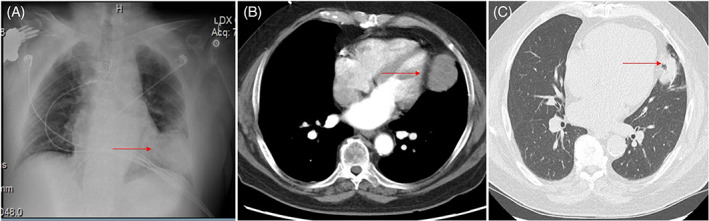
(A) Chest X‐ray showing a rounded mass measuring approximately 8 cm in left lower lobe. (B) CT chest showed a 6.6 cm enhancing soft tissue mass within the left lower mediastinum. A focal point measuring approximately 1 cm can be seen where there does not appear to be a fat plane of separation from the left ventricular myocardium. (C) CT heart angiogram showed a 6.9 by 5.4 cm mass in left inferior mediastinum with a stalk‐like connection to pericardium.
